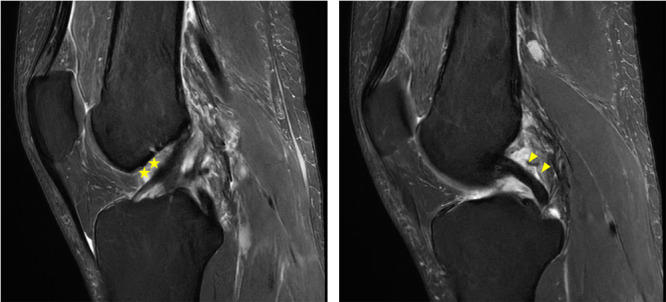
Sagittal fat‐suppressed T2‐weighted MR images of a patient with a partial PCL injury. Depiction of a positive ‘empty roof sign’ (★). The PCL appears continuous (►). ACL, anterior cruciate ligament; MR, magnetic resonance; PCL, posterior cruciate ligament.
